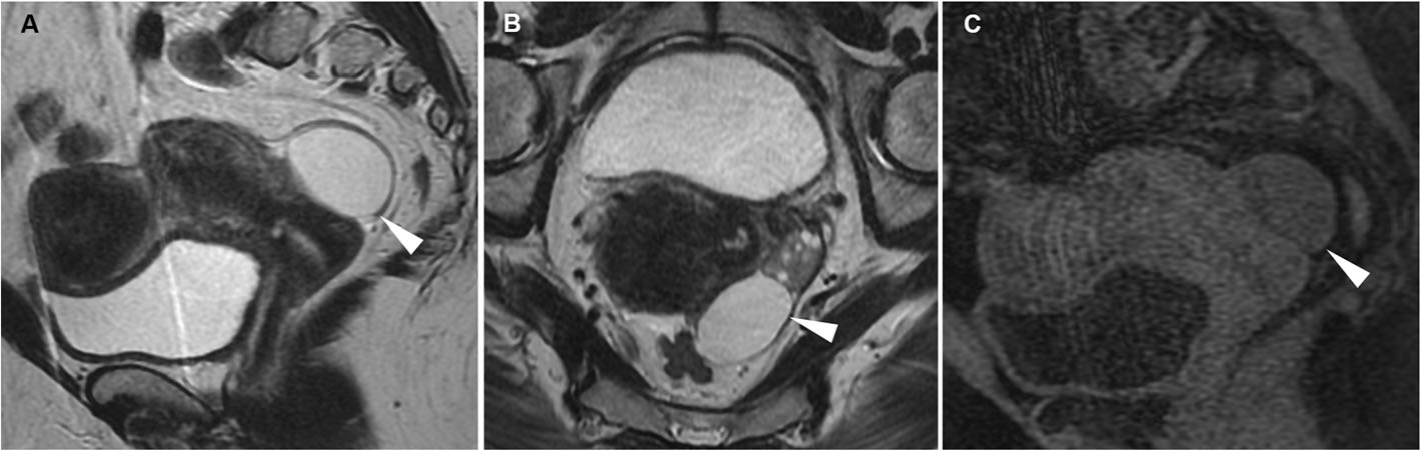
MRI of a functional cyst causing pelvic pain in the periovulatory phase in a 37-year-old woman. Sagittal (a) and oblique-axial (b) T2-weighted images show a 4-cm well-marginated, unilocular cystic lesion with thin walls and homogeneous fluid-like signal intensity arising from the posterior edge of the left ovary (arrowheads), without haemorrhagic hyperintensity on sagittal precontrast fat-suppressed T1-weighted image (c)
